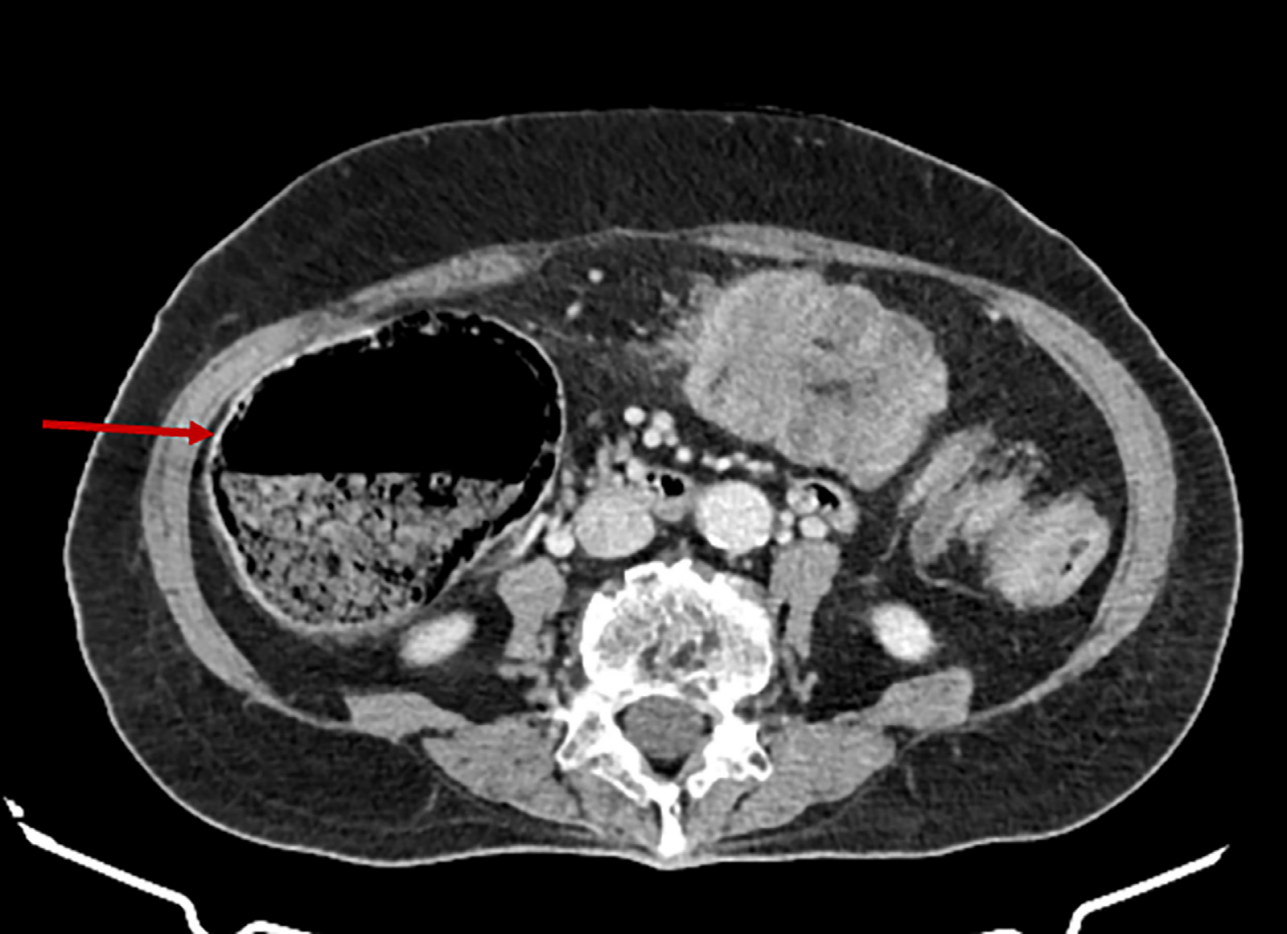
Axial contrast-enhanced CT showing marked cecal distension (red arrow), indicative of upstream colonic obstruction related to the intussusception.
